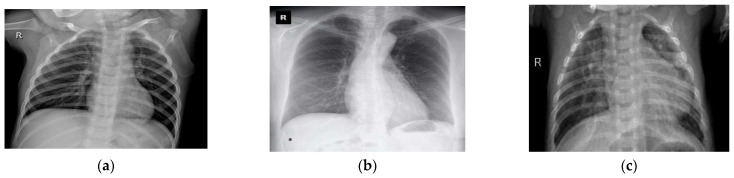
A sample of the experimental chest X-ray dataset: (a) normal or healthy chest, (b) COVID-19, and (c) pneumonia.
